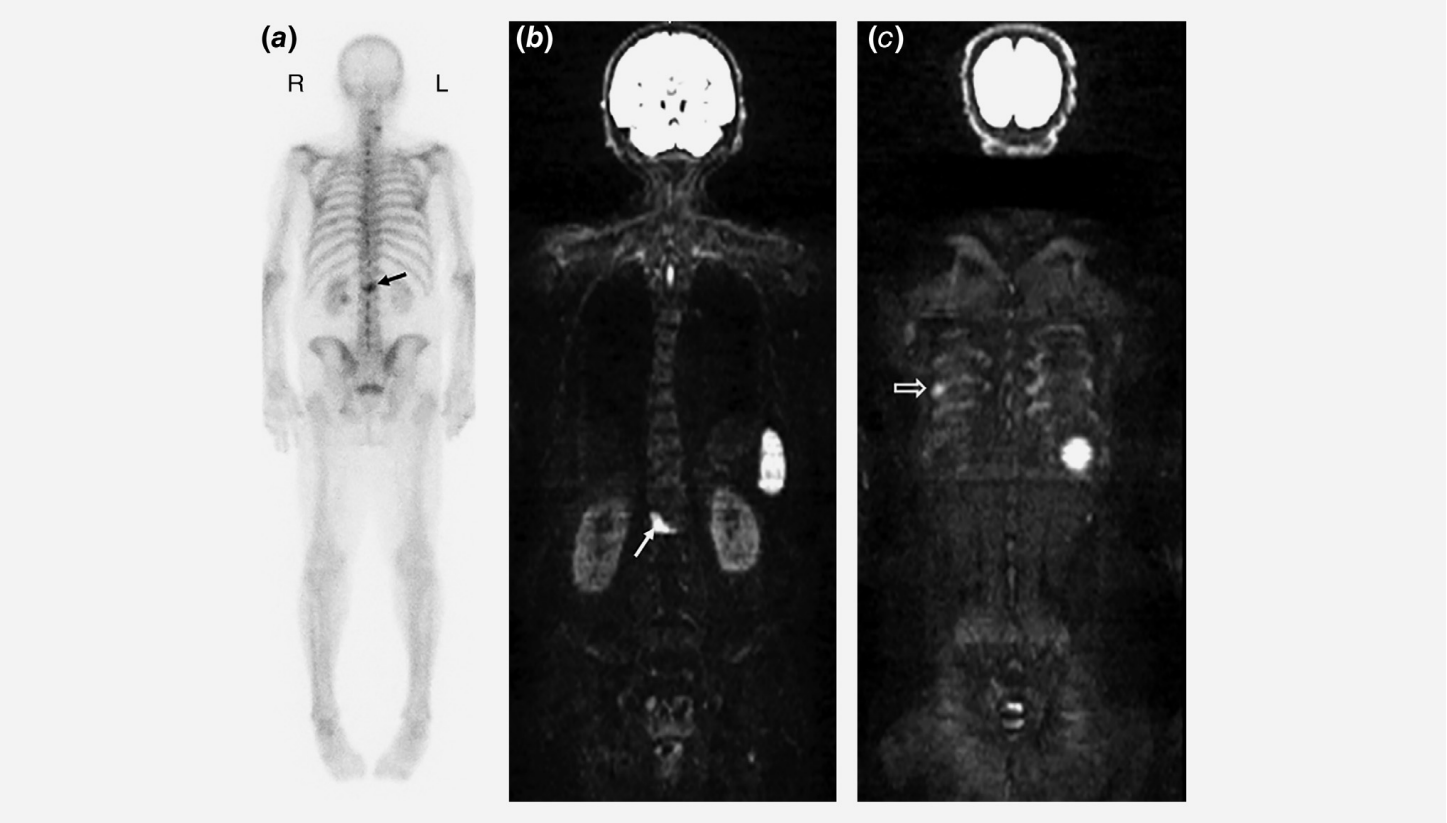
Eighty‐one years. *old male with oligometastatic prostate cancer:* The patient presented 13 years previously with T3a, Gleason 4+4 prostate disease. He was treated with radiotherapy complemented by androgen blockade for 4 years. He experienced biochemical recurrence 10 years post diagnosis. Bone scintigraphy (*a*) showed a solitary metastasis in the lumbar spine (arrow), whole body MRI (*b* = 900 mm^2^/s) confirmed this lesion (*b*, arrow) and revealed the additional rib lesion (*c*, open arrow), indicating the value of utilizing the most accurate imaging modality at the outset prior to planning management.
